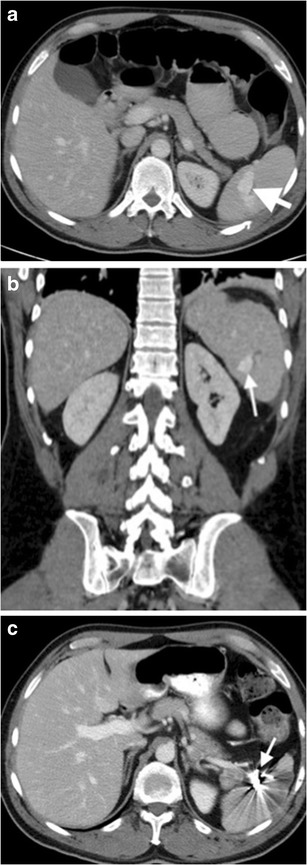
Splenic artery pseudoaneurysm. (a) Axial and (b) coronal contrast-enhanced CT images of a 45-year-old man with a history of a motor vehicle accident demonstrate a saccular-shaped contrast material filling (*arrows*) in the spleen parenchyma representing pseudoaneurysm of splenic artery. (c) Axial contrast-enhanced CT image reveals embolized splenic artery segment (*arrow*) in the splenic hilum
